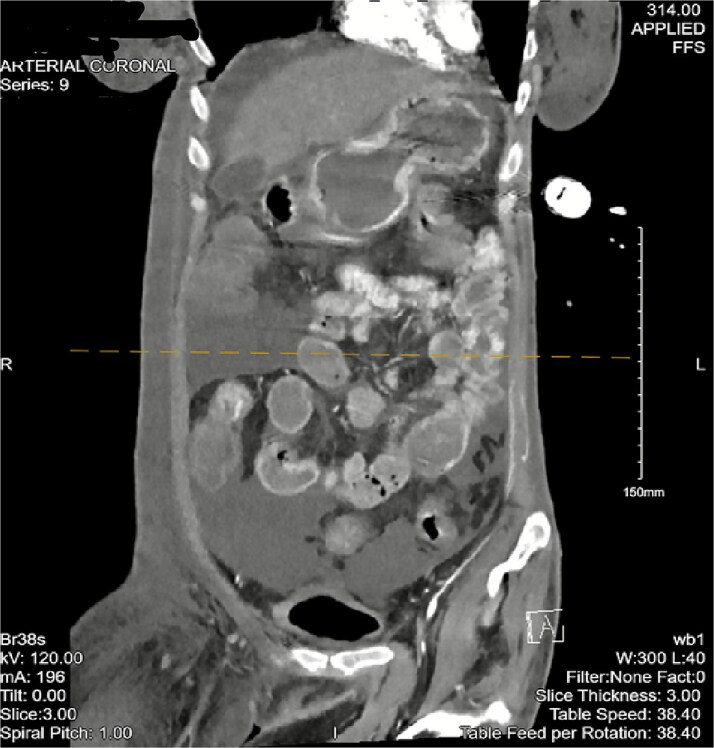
CT angiogram of the abdomen and pelvis—arterial phase with a coronal cross section. Patchy contrast extravasation is observed in the ileocecal region with adjacent distal wall-thickening. Distended loops of small bowel with mesenteric fat stranding are observed. Significant abdominopelvic free fluid is seen, correlating with ascites. Enlarged mesenteric lymph nodes are observed.
